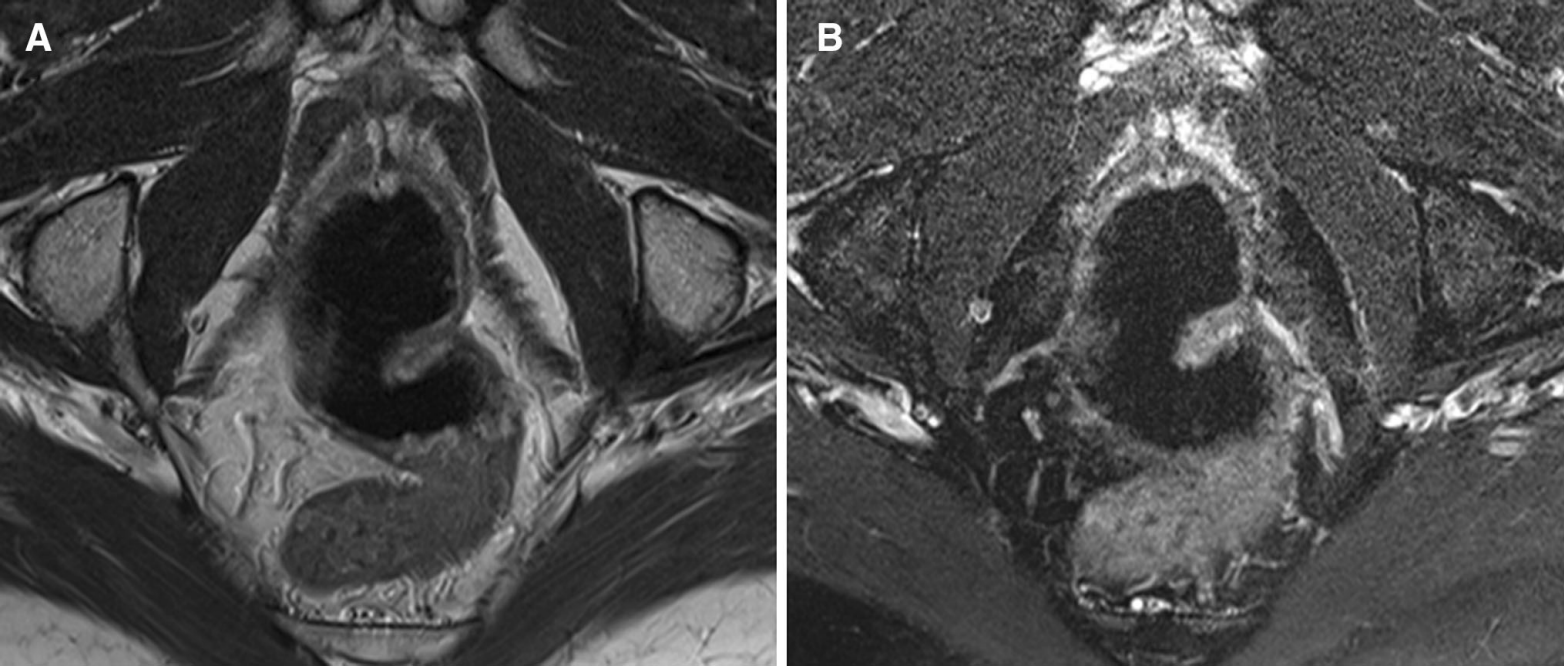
A 45-year-old male with Crohn’s disease and no signs of proctitis at endoscopy. A Axial oblique T2-weighted image and B axial oblique fat-saturated T2-weighted image obtained at the same level shows a moderate increase of T2 signal intensity of the rectal wall
